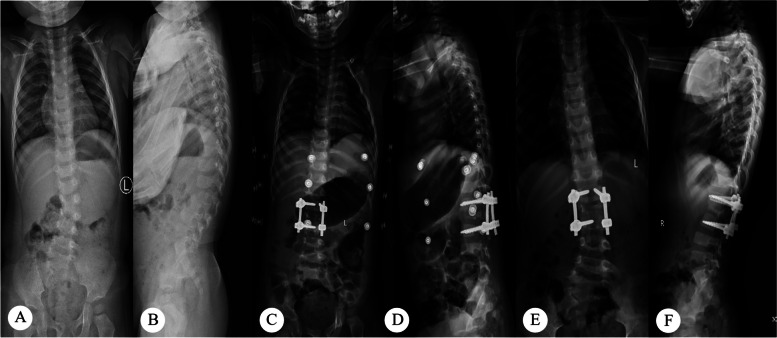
The patient was male, 2.5 years old at the time of operation, L2 hemivertebra. A, B Preoperative anteroposterior and lateral radiographs; C, D Postoperative anteroposterior and lateral radiographs indicated that the patient was almost completely corrected; E, F Anteroposterior and lateral radiographs at the last follow-up, indicating that the Cobb Angle of the main curve progressed to 22°, the spine presented an S-shaped curve, no postoperative kyphosis, no internal fixation dislocation or fracture, and the Cobb Angle of the segment was well corrected
